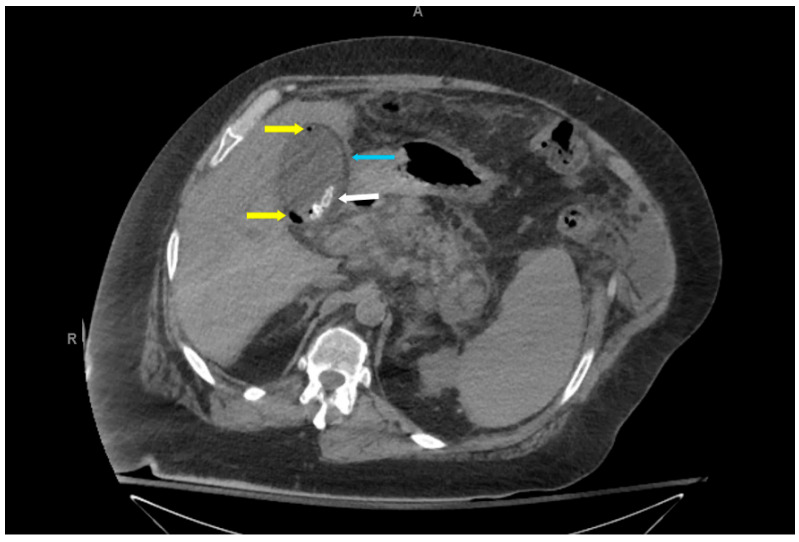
Axial non-contrast computed tomography abdomen (slice thickness 3 mm; soft tissue window, WW 350, WL 40) of a 61-year-old diabetic male patient with emphysematous cholecystitis presenting with right upper quadrant pain. Cholelithiasis (white arrow), foci of air in the gallbladder lumen and wall (yellow arrows), and gallbladder wall edema (blue arrow) were incidentally noted.
